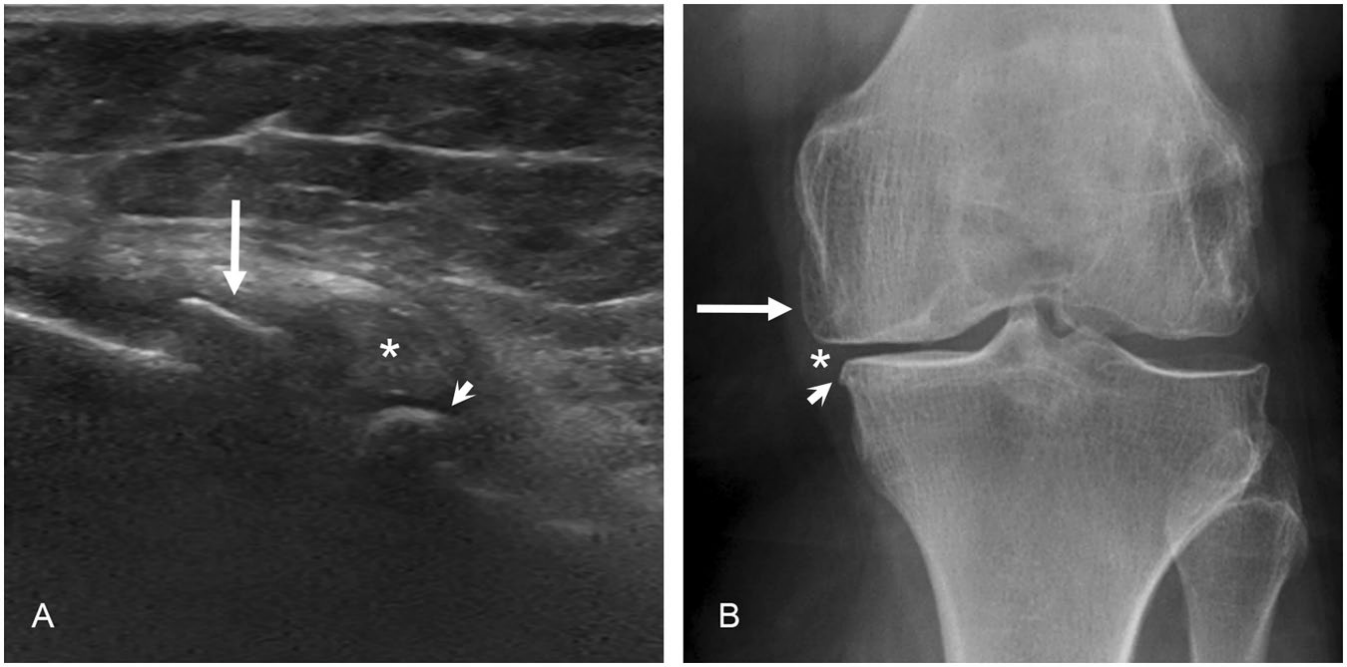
The ultrasonography (US) and radiography of the medial compartment of the knee. (A) US image shows a significant osteophyte on the medial-femoral (arrow) and medial-tibial (arrowhead) sites. (B) On the corresponding radiography, no corresponding osteophyte is detected on the medial-femoral site (arrow), whereas only medium osteophyte is seen on the medial-tibial site (arrowhead). The asterisks describe the dislocation of the medial meniscus to the joint space.
